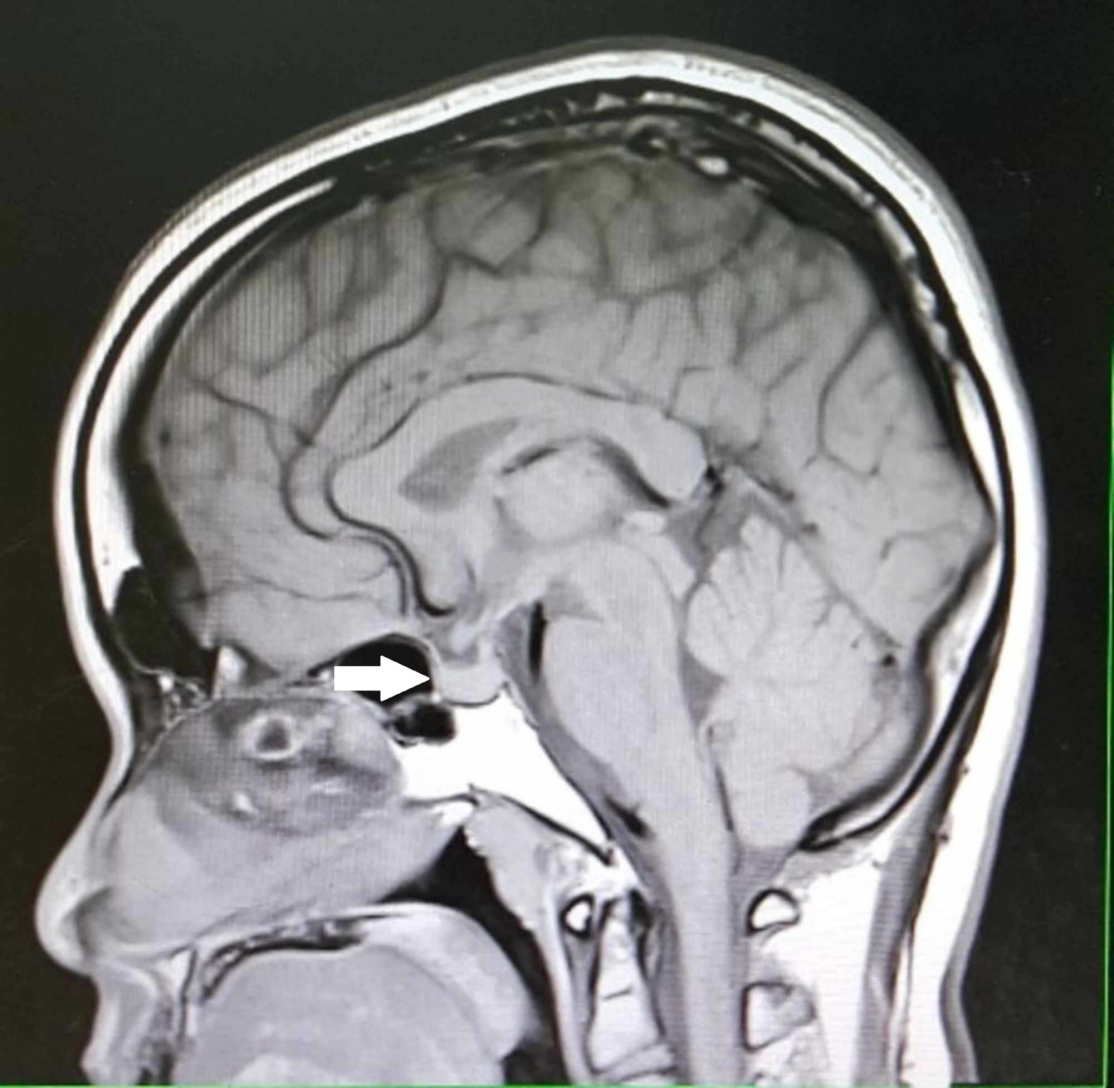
T1-weighted sagittal MRI repeated after one month revealed a shrinkage of pituitary mass
